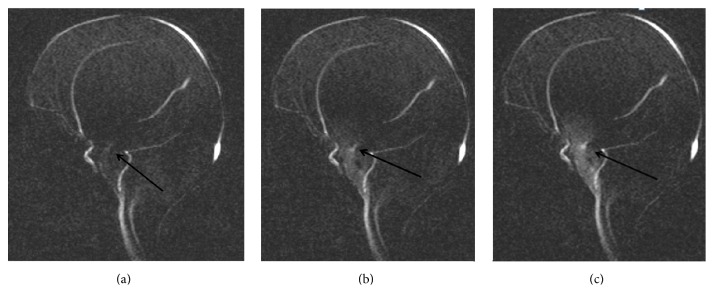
(a, b, c) MRI CSF flow study: arrow shows CSF flow at the floor of third ventricle.
